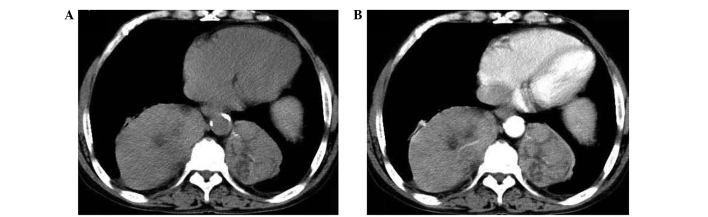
Unenhanced thoracic computed tomography (CT) scan showing (A) multiple well-marginated bilateral solid masses, without calcification, located at the paravertebral region, and (B) lesions showing uneven enhancement during the arterial phase. The necks of the ribs were extremely thin.
